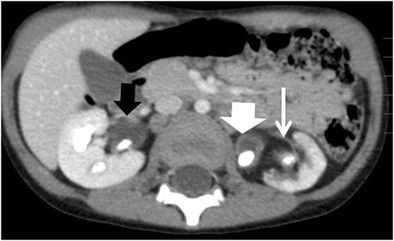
Diffuse XPN. Contrast-enhanced nephrogenic phase transverse CT shows bilateral kidney lithiasis. Right kidney presents a dilated pelvis with a stone inside, while left kidney shows a stone in the pelvis with fatty proliferation (*thin white arrow*) and an obstructive stone inside a dilated ureter (*thick white arrow*)
